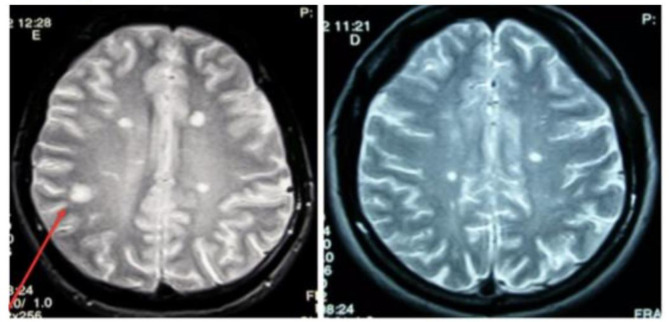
Juxtacortical lesion on MRI.
